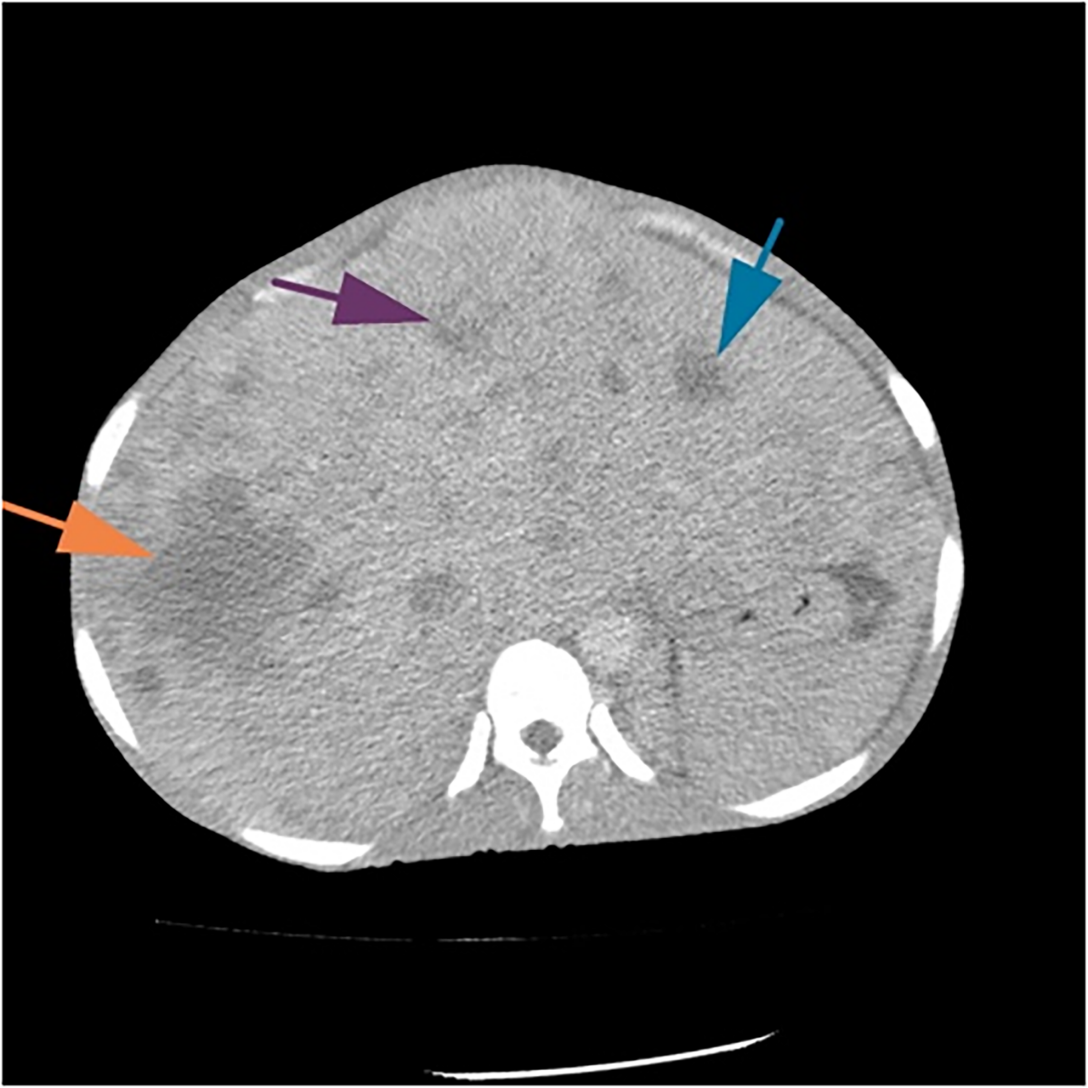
Axial computed tomography images of the abdomen, at the level of the liver. The image shows innumerable hypodense minimally enhancing lesions (arrows), consistent with metastasis.
